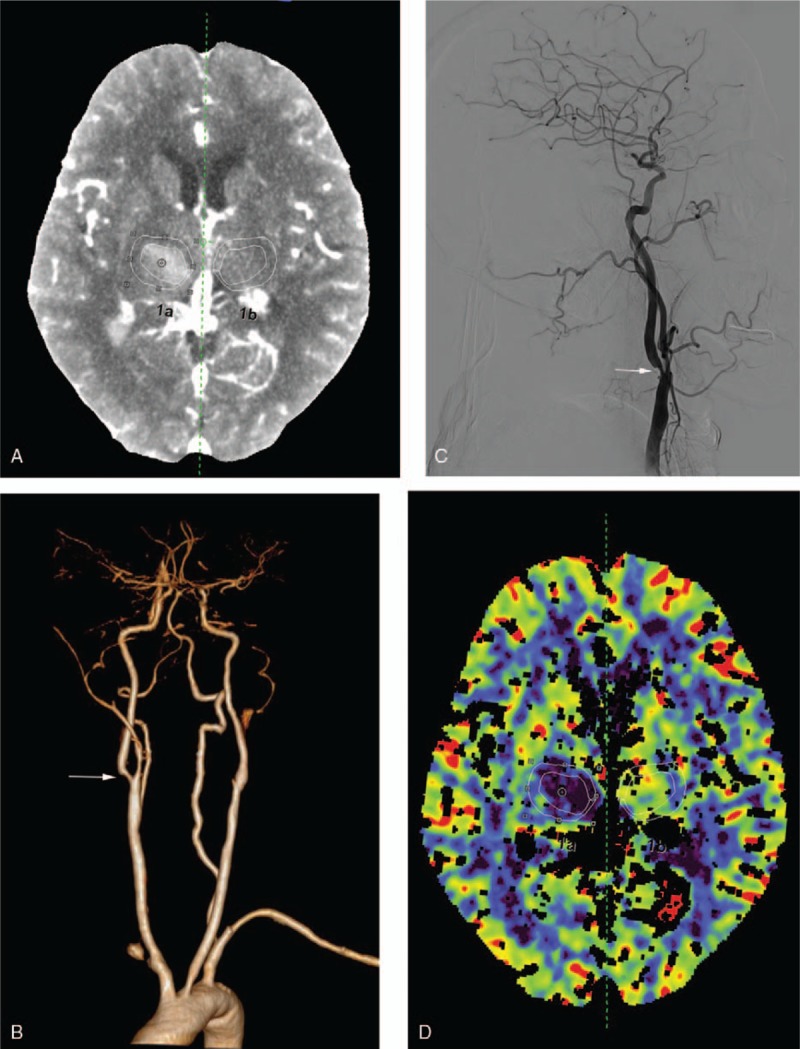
A 58-year-old female was diagnosed with right basal ganglion hemorrhage by NCCT (A). CTA (B) and DSA (C) identified the 62% stenosis of right internal carotid artery with white arrow. The CTP showed the mean CBF of perihematoma (20.24 mL/100 mL/min) and contralateral homologous region (48.05 mL/100 mL/min).
